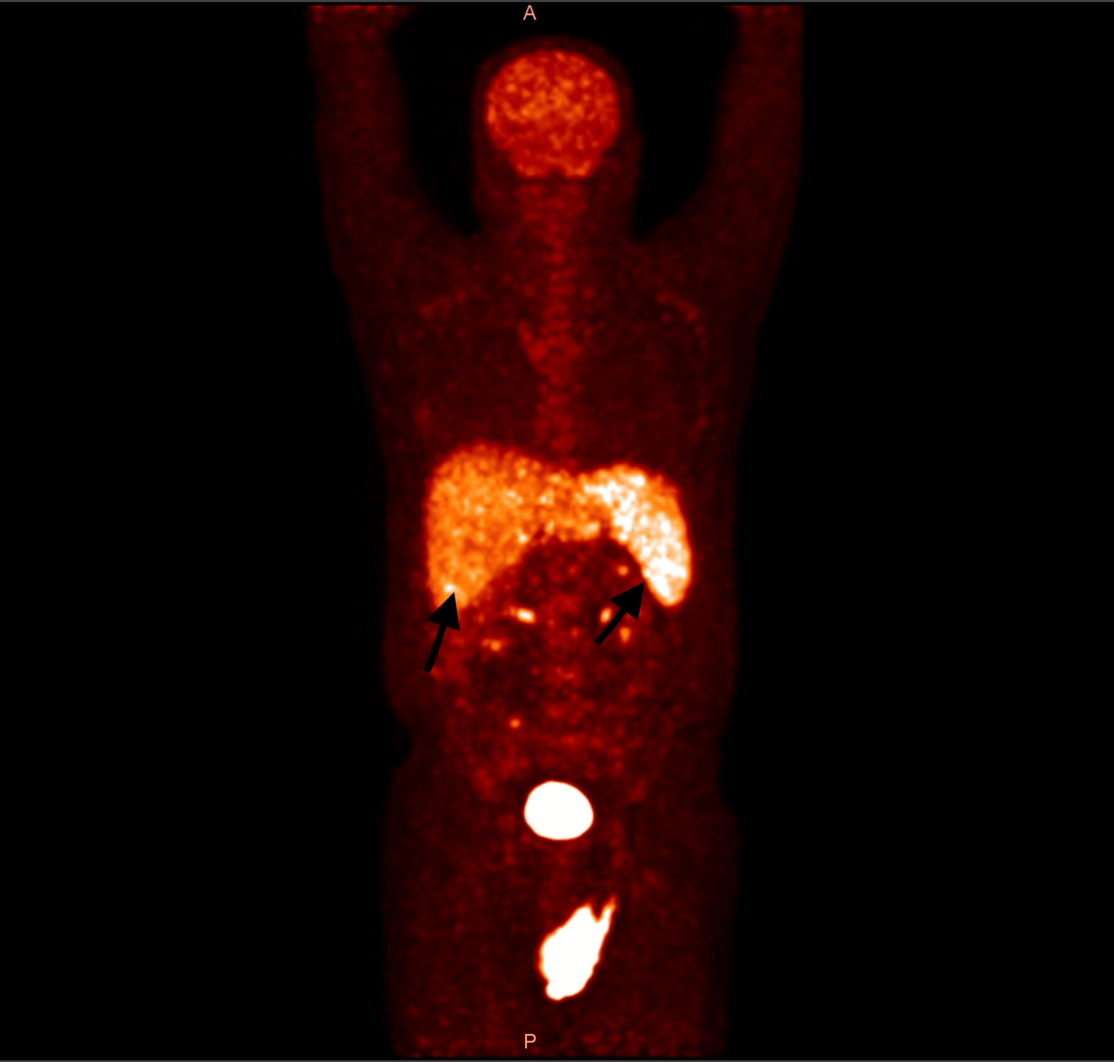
Whole-body PET-CT (coronal view) Diffuse FDG uptake is noted in the enlarged liver and spleen, without evidence of focal lesions or lymphadenopathy. FDG, fluorodeoxyglucose
